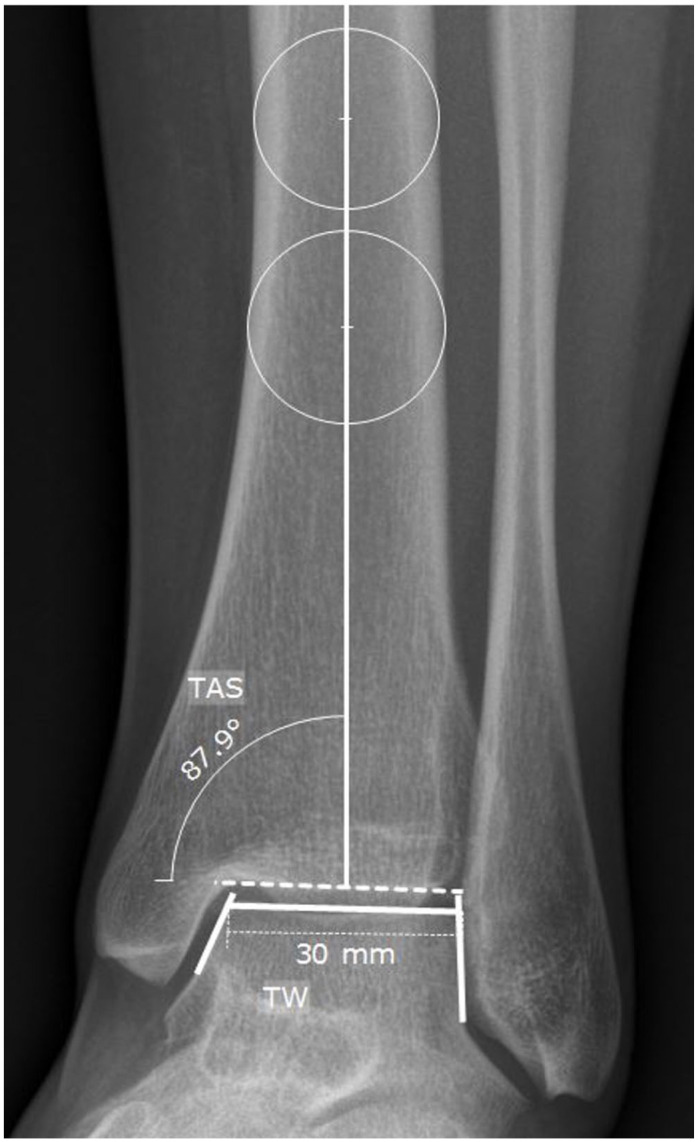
Measurements of tibial anterior surface (TAS) angle and talar width (TW) on an anteroposterior weightbearing radiograph: the TAS angle is the angle between the tibial axis and distal tibial articular joint surface measured in degrees. For the TW, a tangent line is drawn along the medial and lateral talar shoulder. Another tangent line is drawn on the joint surface of the talar dome. The TW is the distance of the 2 intersections of these lines, measured in millimeters.
